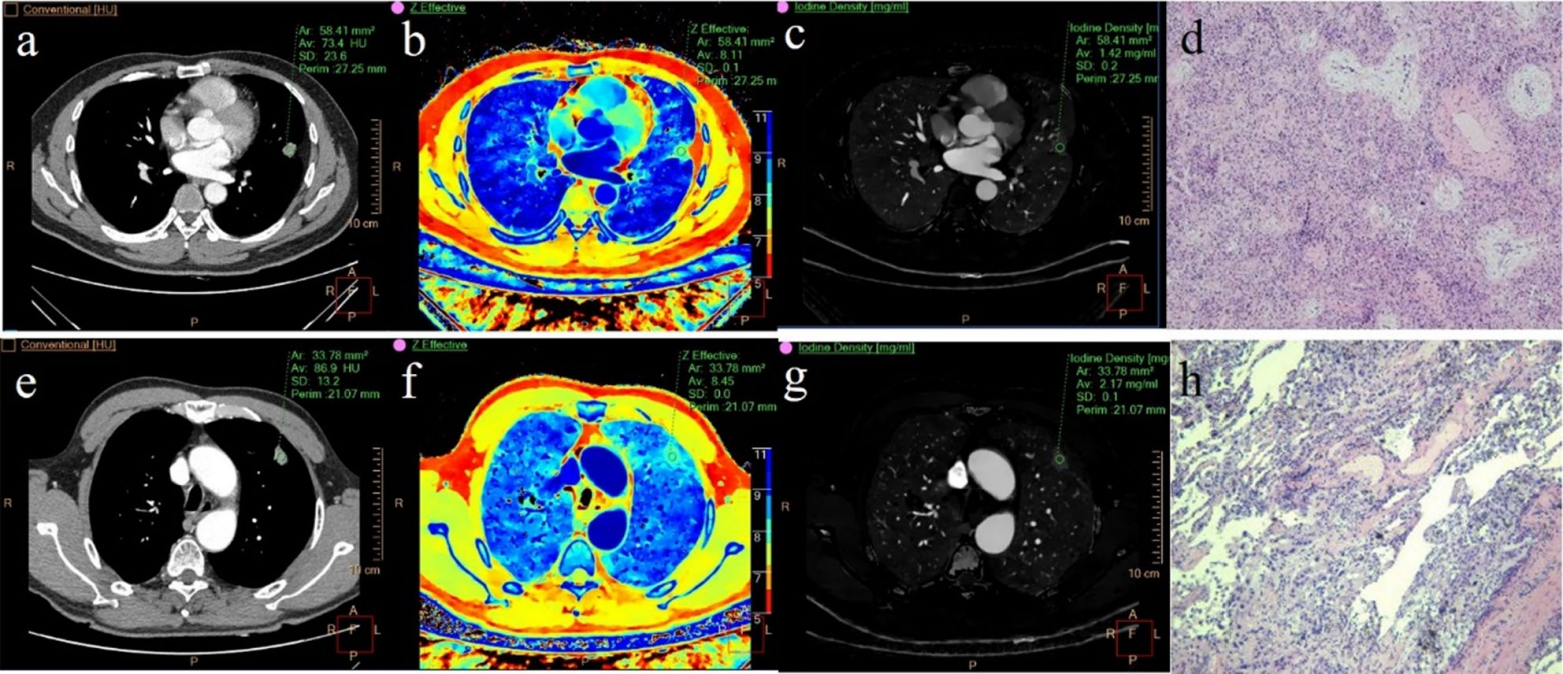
A 41-year-old male patient with inflammatory pseudotumor in the upper lobe of the left lung (a–d) and a 64-year-old male patient with lung adenocarcinoma (e–h), both pathologically confirmed. a and e are enhanced phase CT images, and the CT values of the lesions are 73.4 HU and 86.9 HU respectively; (b, f) are Zeff images with Zeff values of 8.11 and 8.45 respectively; (c, g) are iodine images, and the IC values are 1.42 mg/ml and 2.17 mg/ml respectively; (d, h) are postoperative pathological pictures.
